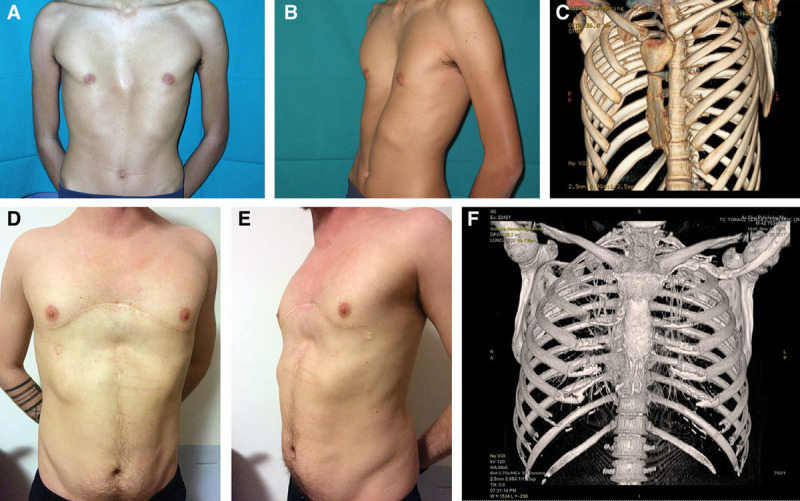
Case 2: A 26-year-old man showing severe pectus deformity with limited functional impairment. A and B, Preoperative view. C, Preoperative 3D CT scan of the chest. D and E, Late postoperative view showing stable correction. F, 18-month follow-up 3D-CT scans after correction.
